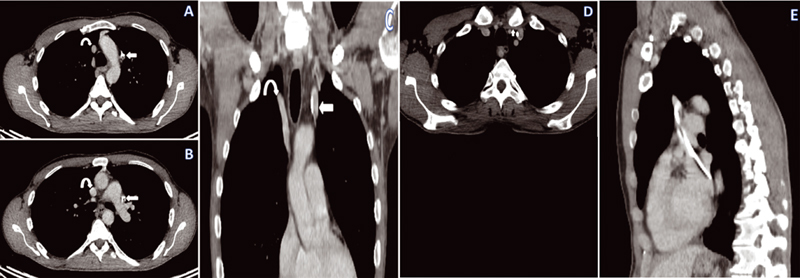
Computed tomography scan. (A, B) Axial view demonstrating the catheter in the persistent left superior vena cava and the right vena cava (curved arrow). (C) Coronal view demonstrating the catheter in the persistent left superior vena cava (arrow) and the right vena cava (curved arrow). (D) Axial view showing the left brachiocephalic vein that communicates the persistent left superior vena cava and the right superior vena cava. (E) Axial view demonstrating the left superior vena cava draining into the coronary sinus.
